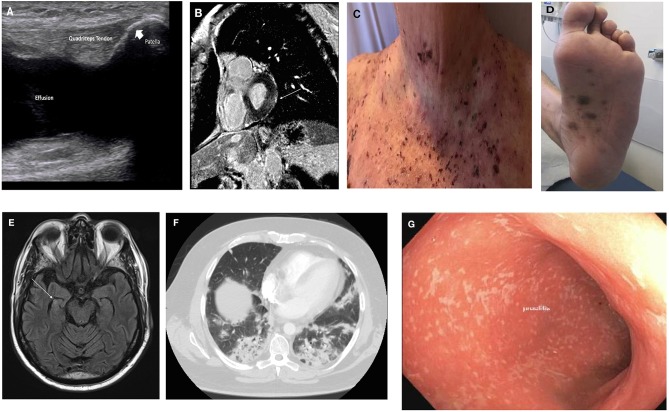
Clinical manifestations of irAE. (A) Inflammatory arthritis: US image of knee effusion; (B) myopericarditis: cardiac MRI with late gadolinium enhancement overlying basal left ventricular lateral wall (arrow); (C) bullous pemphigoid; (D) lichenoid dermatitis; (E) encephalitis: brain MRI with hyperenhancement of right hippocampus (arrow); (F) pneumonitis: chest CT with bilateral lower lobe infiltrates; (G) colitis: endoscopic findings of pan-colitis.
